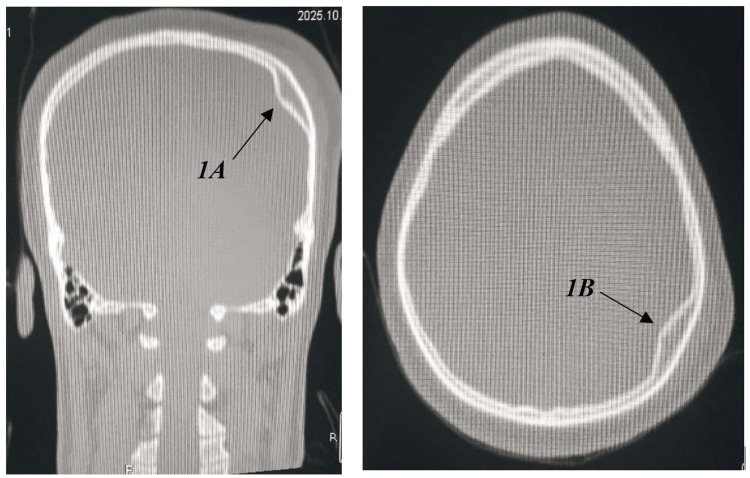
(A) Coronal and (B) Axial non-contrast cranial computed tomography (CT) scans (bone window) revealing a focal depressed fracture limited to the inner table of the left parietal bone (arrows). Note the complete preservation and intactness of the outer table and diploic space.
